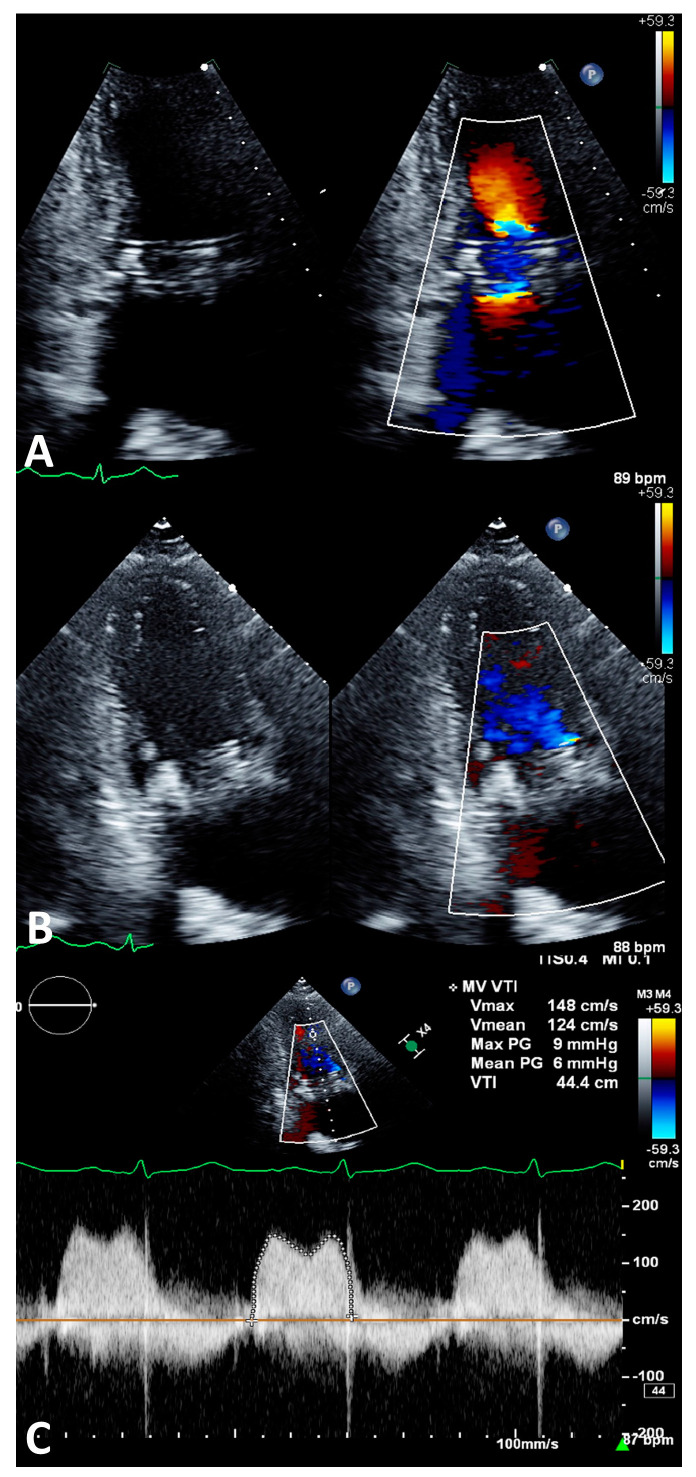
(A–C) shows postprocedural transthoracic echocardiographic images following ViV TMVR. (A) shows a 2-chamber color-compare image in early diastole with mild flow acceleration across the transcatheter mitral valve. (B) shows a 3-chamber color-compare image in early systole without significant MR or LVOT flow acceleration appreciated. (C) shows a mean transmitral gradient of 6 mmHg at a heart rate of 87 beats per minute.
